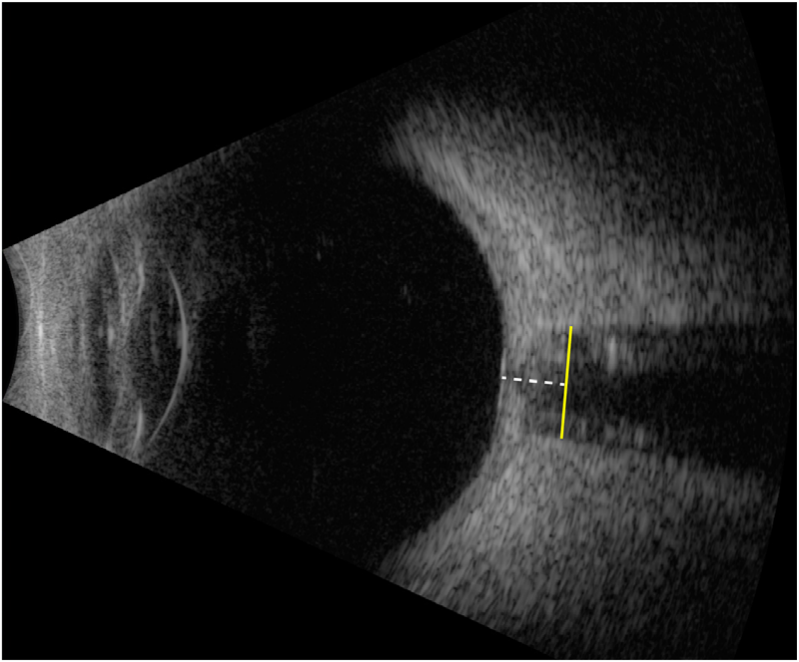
Ultrasonography measurement of optic nerve sheath diameter. Vertical axial B-scan demonstrating good centration of the optic nerve and distinct optic nerve sheath boundaries. ONSD (*yellow line*) was quantified 3 mm (*white dashed line*) posterior to the globe.
